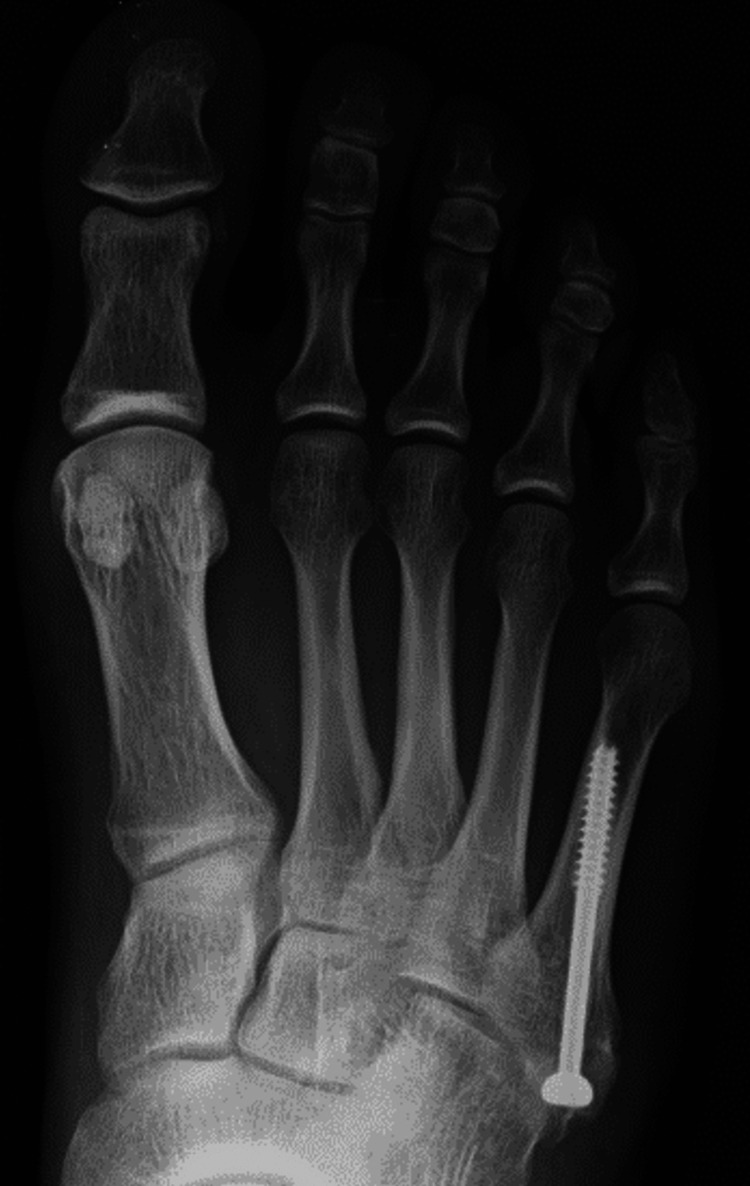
Plain radiograph at the 16-month follow-up visit showing healed and remodeled second and third metatarsals.
